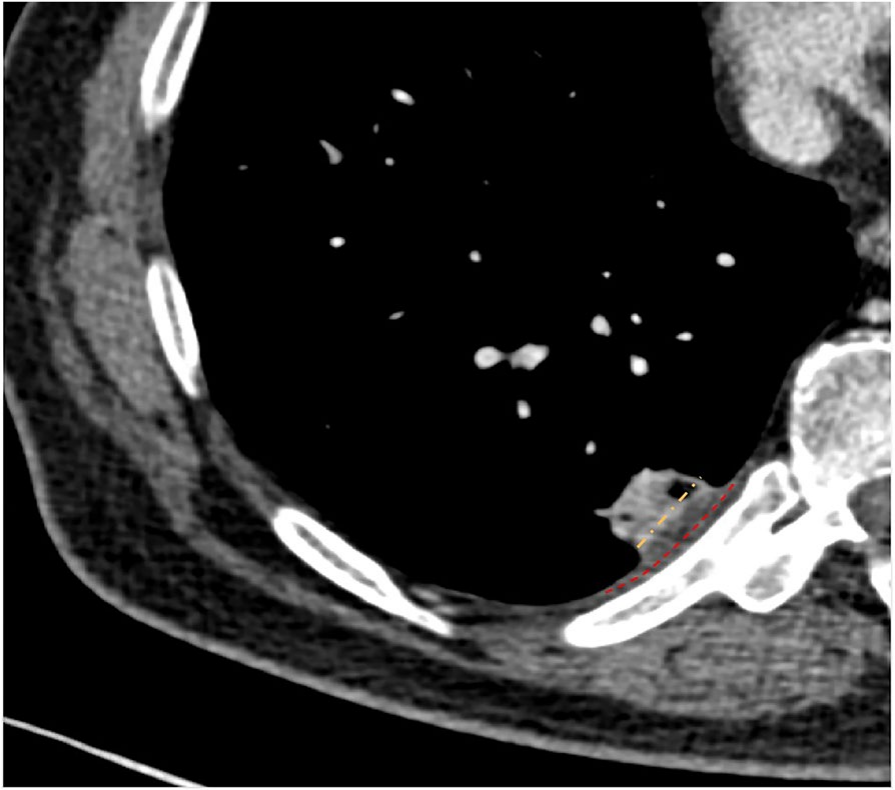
SPAN with pleural thickening and type II of nodule-pleura relationship. A 53-year-old man with right lower lobe SPAN which is confirmed as an inflammatory lesion. On the axial enhanced CT image, it is found that there is a visible increase in pleural thickness (red dashed line) beyond the normal pleural appearance adjacent to the nodules (pleural thickening). R-thickening is defined as the ratio of the length of adjacent thickened pleura (red dashed line) to nodule diameter (yellow dashed line). Its R-thickening is 1.39. The nodule-pleura relationship is type II (nodule clinging to the thickened pleura without pleural infiltration)
